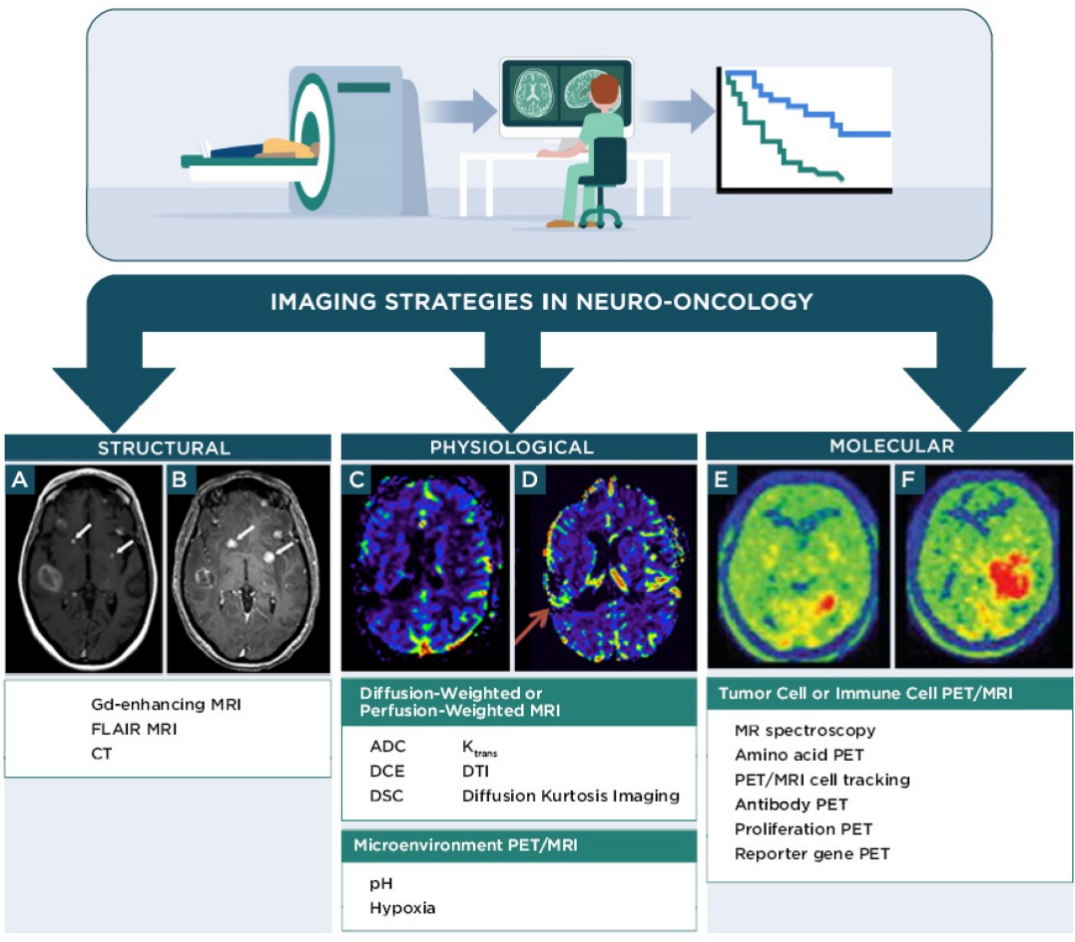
Overview of imaging techniques used to predict response to immunotherapy in neuro-oncology research. A, B: gadolinium-enhanced *T_1_* MRI images in a patient with melanoma brain metastases before (A) and 11 days after (B) treatment with pembrolizumab checkpoint immunotherapy. Arrows indicate resected lesions, which showed inflammatory cell responses attributed to treatment effects rather than disease progression. (Adapted with permission from 46 copyright 2015 American Association for Cancer Research) C,D: Perfusion weighted MRI images of relative cerebral blood volume in a patient with relapsed malignant glioma at 1.5 months (C) and 10.5 months (D) after treatment with dendritic cell vaccine immunotherapy. The arrow in D indicates increased relative cerebral blood volume in the region of the tumor attributed to progressive disease at the latter time point. (Adapted with permission from 47 copyright 2010 Springer Nature) E,F: [^11^C]MET amino acid PET images in a patient with recurrent GBM before (E) and 12 weeks after (F) treatment with WT1 peptide vaccine immunotherapy. Increased PET signal (red) is attributed to disease progression. (Adapted with permission from 48 copyright 2012 American Association of Neurological Surgeons) DCE, dynamic contrast-enhanced MRI; *K*_trans_, rate of transfer from plasma to extravascular space in perfusion MRI; DSC, dynamic susceptibility-weighted contrast-enhanced MRI; ADC, apparent diffusion coefficient in diffusion MRI; DTI, diffusion tensor imaging MRI; DKI, diffusion kurtosis imaging MRI.
